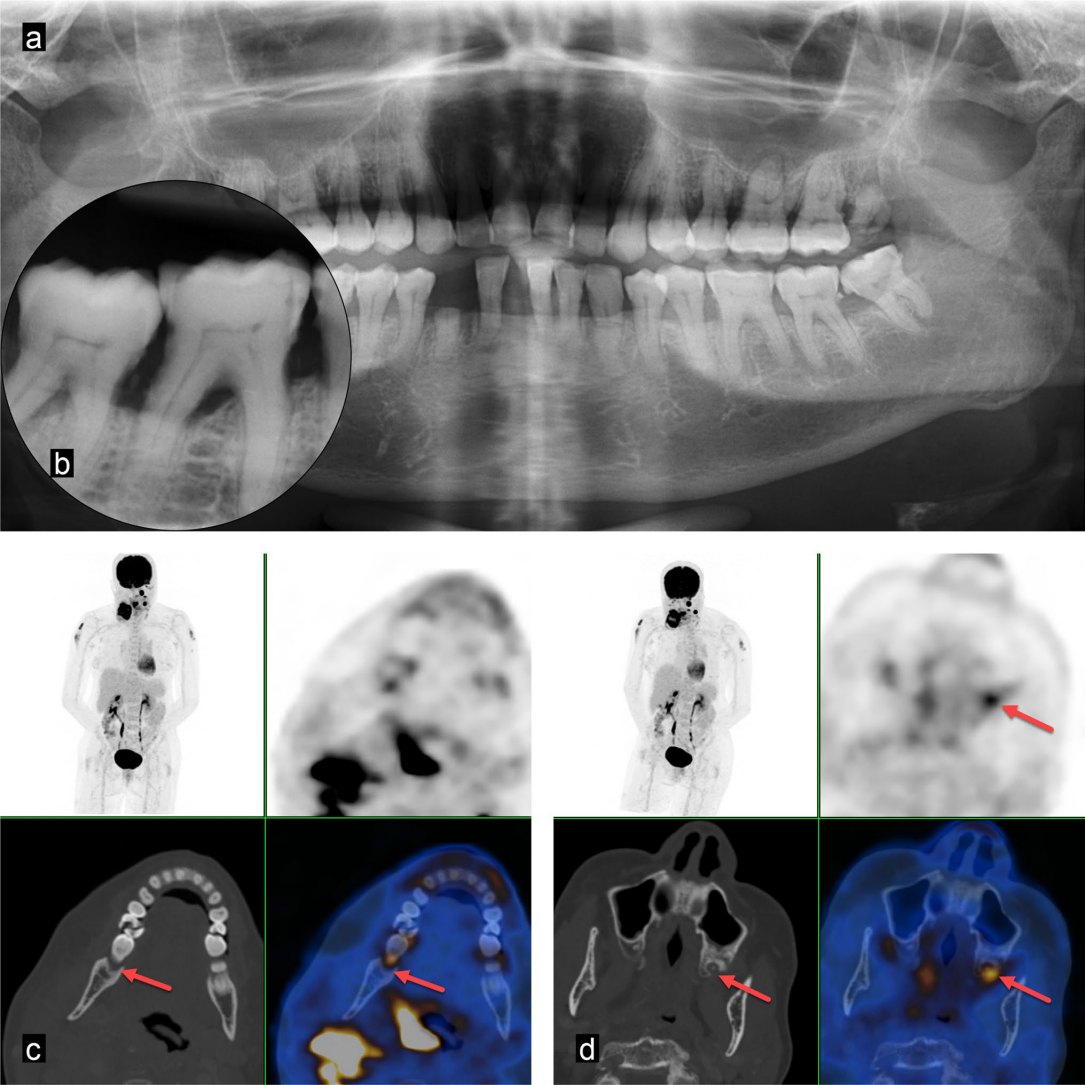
A 66-year-old woman with right-sided hypopharyngeal carcinoma cT4b cN2c M0. Clinical examination showed severe generalised chronic periodontitis and several heavily decayed teeth due to caries and poor oral hygiene. Two out of several metabolically active pathologies are displayed here. a Panoramic radiography shows several remaining tooth roots, calculus, and a reduced alveolar bone level. b The periapical radiograph of tooth 47 visualises a marginal periodontitis. c, d FDG-PET/CT demonstrates tooth-related pathologies with increased metabolic activity (SUV_max_ 13.8 for tooth 28 and SUV_max_ 5.0 for tooth 47, red arrows). All non-preservable teeth were extracted, preservable teeth restored, dental hygiene performed, and fluoride splints prepared
